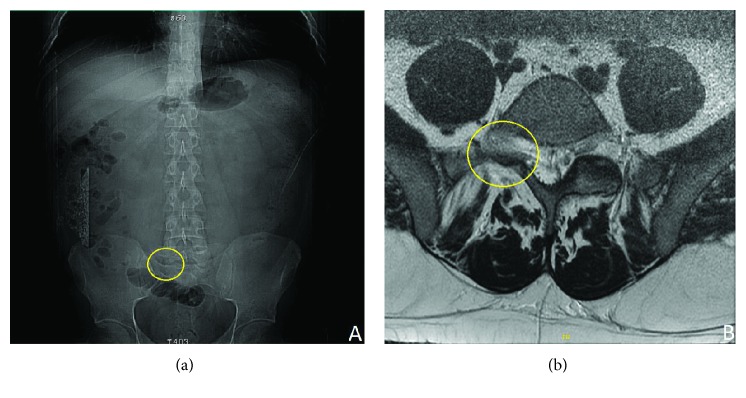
(a) Scout X-ray image showing the absence of the right L5-S1 zygapophyseal joint. (b) On axial spine MRI, the right articular process of S1 is absent, with the inferior articular process of the L5 markedly hypoplastic.
